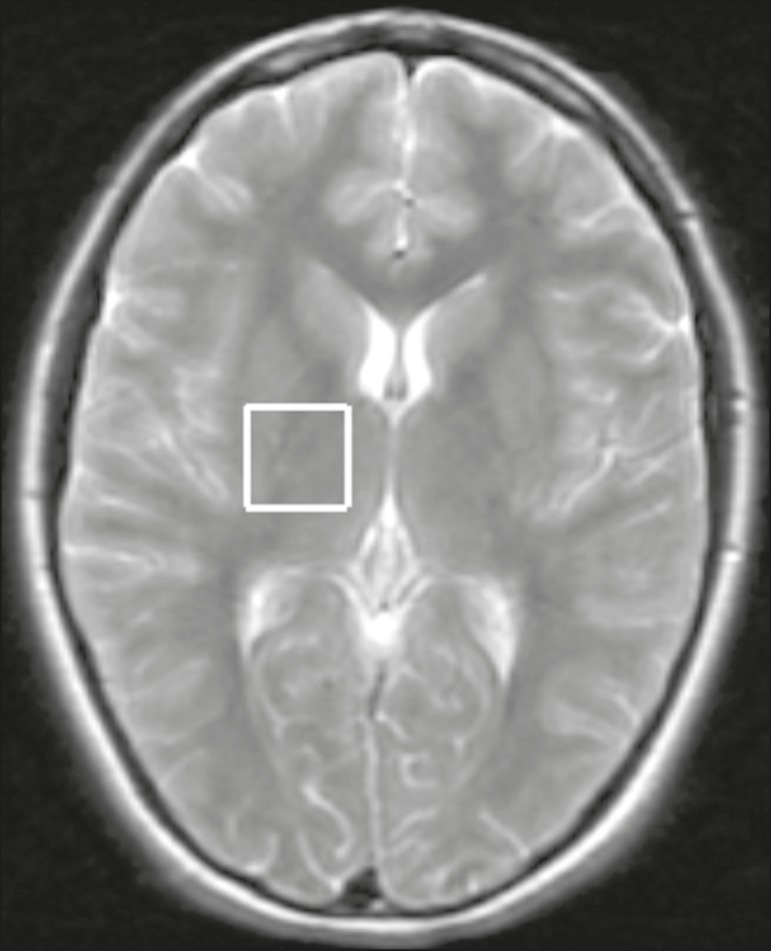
Axial T2-weighted image showing the MRS VOI located on the right
